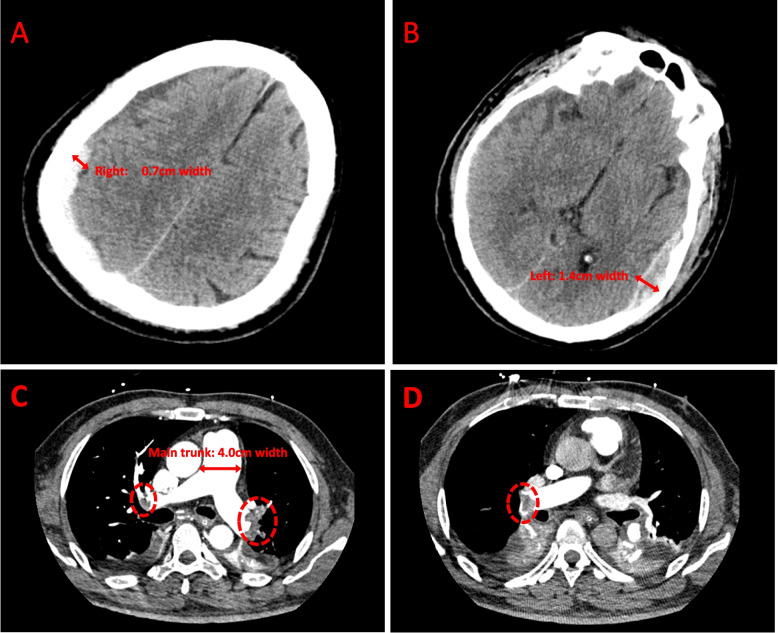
Head CT and CTPA on 15th May. A A crescent-shaped high-density shadow under the inner plate of the right top skull, indicating a right subdural hematoma, the widest part of which is about 0.7cm; B The left temporal fusiform mixed high-density shadow, indicating the left epidural hematoma, the widest hematoma about 1.4cm; C and D show pulmonary arteries embolized at different levels of the lungs, Pulmonary artery thickening, the maximum width of the main trunk is about 4.0cm, the left and right pulmonary trunk distal and branches filled with multiple defects, suggesting pulmonary embolism. (Note: The places marked in the circle are thrombus of the pulmonary artery)
